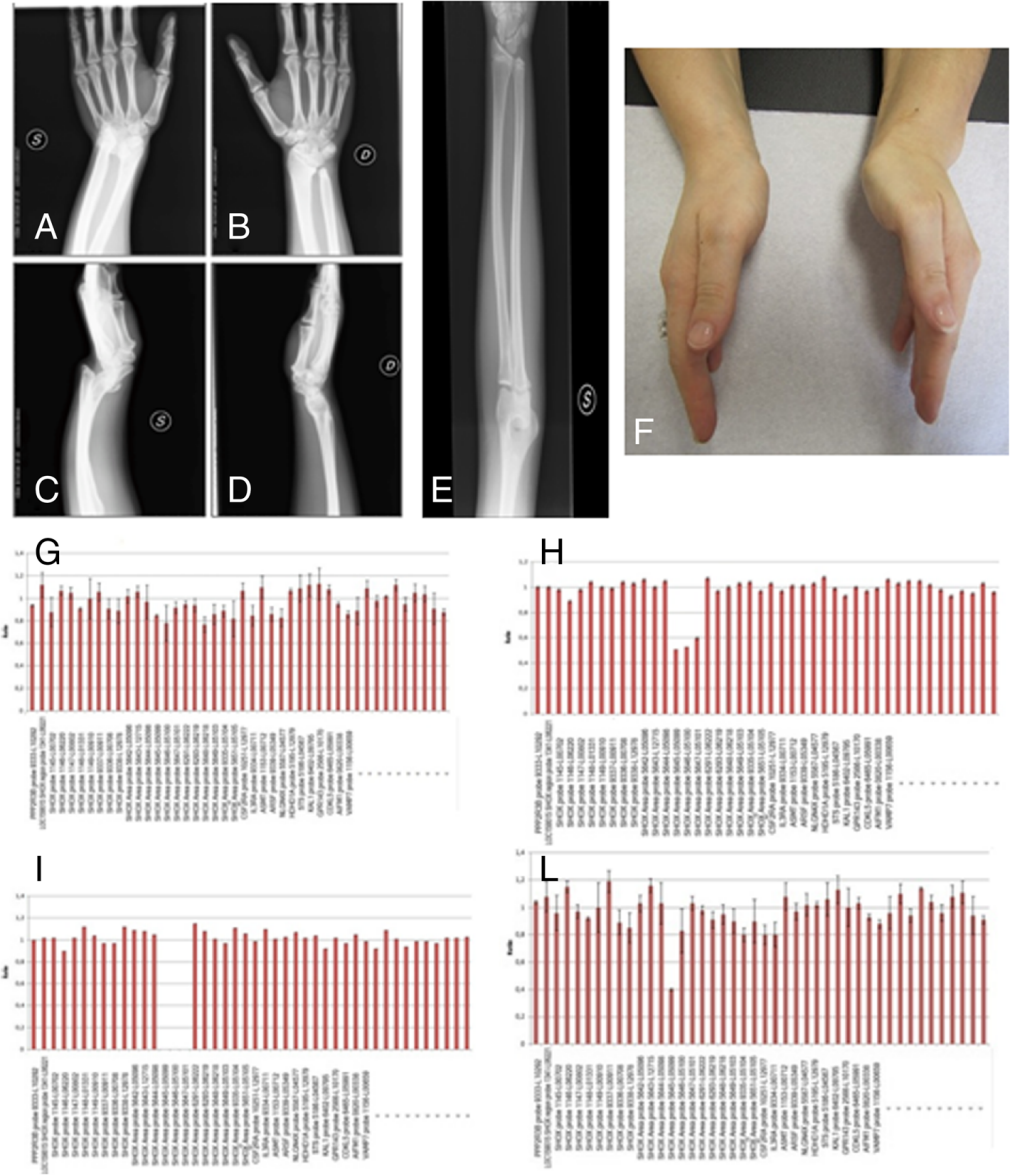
Female 39-year-old: Bilateral forearm radiographs show triangularization of distal epiphysis and bowing of diaphysis of radius bilaterally; distal radio-ulnar articulations appeared radiographically lucent. Palmar subluxation of the left carpus (A-D). Female 6-year-old: Left forearm radiography shows triangularization of distal epiphysis and bowing of diaphysis of radius, radiolucency of distal radio-ulnar articulation (E). Madelung deformity of family 3 index case (n.8) (F). The lower panel showes MLPA assay results. (G) Control: Normal peaks were classified as showing a ratio of 0.65-1.35 (H) Heterozygous deletion of the 13296-L15336, 05645-L05099 and 05646-L15507. MLPA probes have a ratio < 0.65. (I) Homozygous deletion of the 13296-L15336, 05645-L05099 and 05646-L15507 MLPA probes showing a ratio = 0. (L) Heterozygous deletion of the 05645-L05099 MLPA probes.
